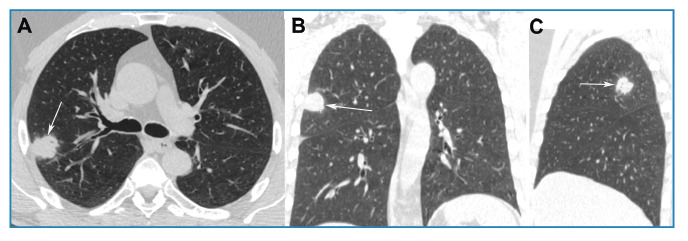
Control chest CT images with axial (A), coronal (B), and sagittal (C) reconstruction, obtained 6 months after those in Figure 1, demonstrate nodule growth, with a diameter of approximately 25 mm (arrows).
